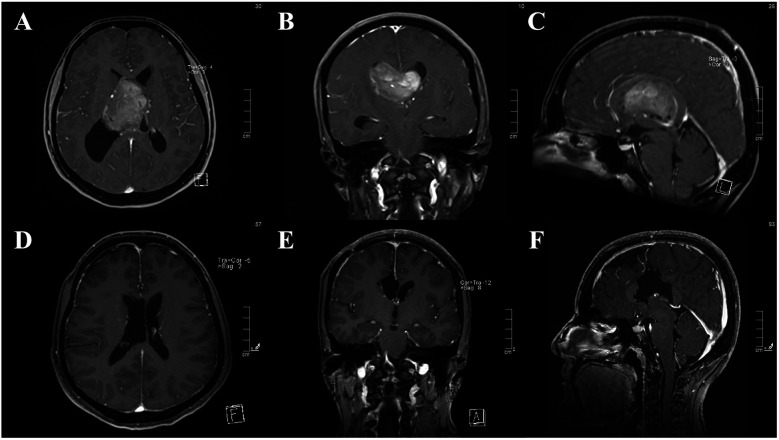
(A–C) Pre-operative contrast-enhanced MRI images of central neurocytoma located in both lateral ventricles. (D–F) Post-operative contrast-enhanced MRI images of gross total resection.
